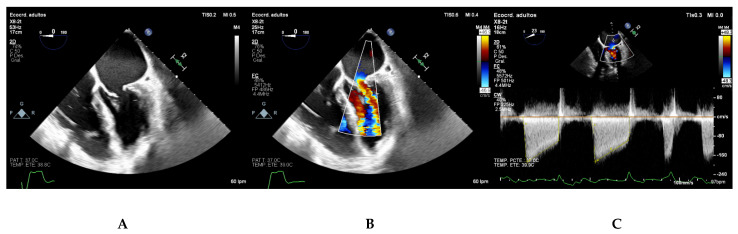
(A) Mid-esophageal four chamber view. (B) Color Doppler at the mitral valve level in a mid-esophageal four chamber view. (C) Continuous wave Doppler parallel to the mitral inflow in mid-esophageal four chamber view.
